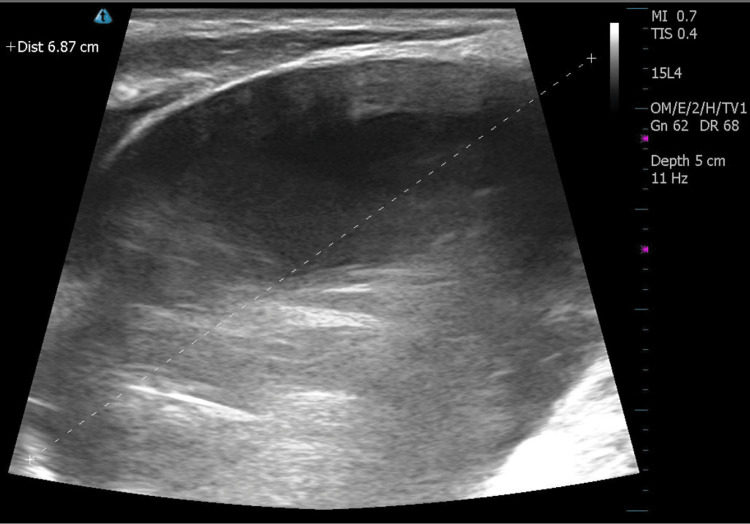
Two-dimensional ultrasound (2DUS) of patient's cervical lymphocele prior to sclerotherapy Two-dimensional ultrasound (2DUS) of the patient’s left neck 16 days following embolization of cervical lymphocele. Transverse orientation shows a large, hypoechoic and fluid-filled mass measuring 6.87 cm.
